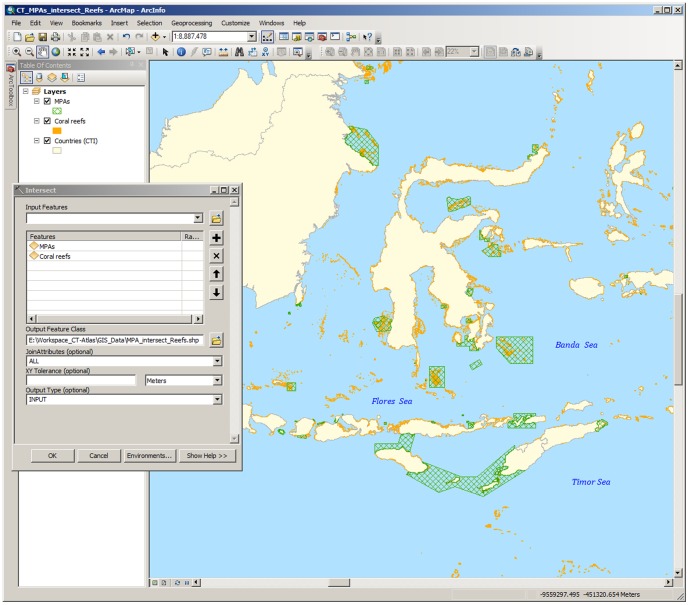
Screen shot demonstrating the overlaying of Marine Protected Area (MPA) and coral reef data from the CT-Atlas to derive the results available online in Table S1.
